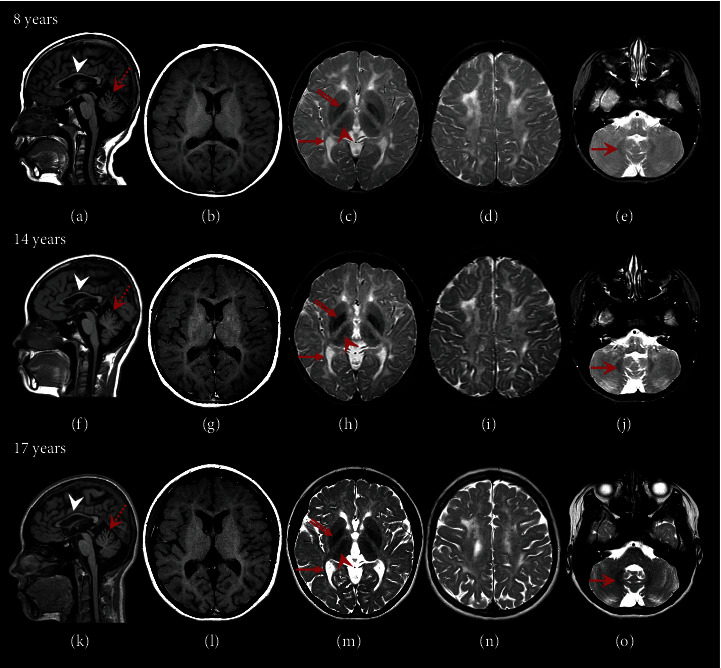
MRI features. Serial neuroimaging at Ages 8, 14, and 17 years, including sagittal T1- (Column 1: (a), (f), and (k)), axial T1- (Column 2: (b), (g), and (l)), and axial T2- (Column 3: (c), (h), and (m); Column 4: (d), (i), and (n); Column 5: (e), (j), and (o)) weighted MRI images. Diffuse hypomyelination is evident: there is hyperintensity of the white matter on T2-weighted imaging (c, d, h, i, m, n), together with a variable signal of the white matter on T1-weighted imaging, with hyperintense/isointense/slightly hypointense areas of the white matter (b, g, l), compared to grey matter structures. Relative myelin preservation (T2-hypointensity) is seen in the optic radiations (red arrows in (c), (h), and (m)), the anterolateral nucleus of the thalamus (red arrowhead), the globus pallidus (red double-lined arrow), and the dentate nucleus (red arrow in (e), (j), and (o)), with overall mildly improved myelination over time. Mild cerebral atrophy is evident by Age 17. A thin corpus callosum (white arrowheads) and mild superior cerebellar vermis atrophy (red dashed arrow) are demonstrated, without significant levels of progression over time (a, f, k).
